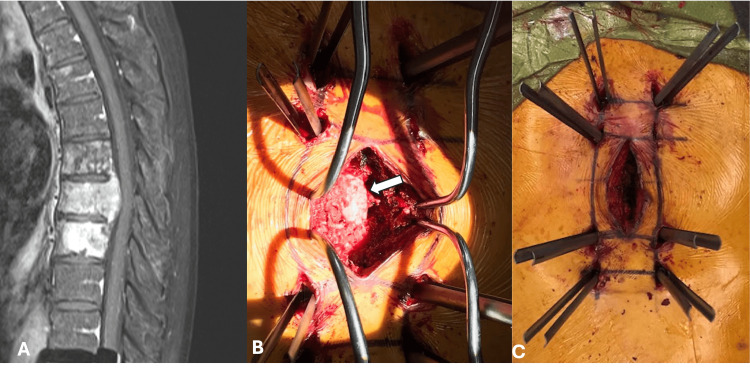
(A) Sagittal T1 MRI with contrast of a patient with metastatic prostate cancer with T8 epidural cord compression, (B) patient was managed with T8 bilateral transpedicular decompression with ligation of T8 nerve root (arrow), partial corpectomy, and (C) T6-T11 percutaneous instrumentation.
